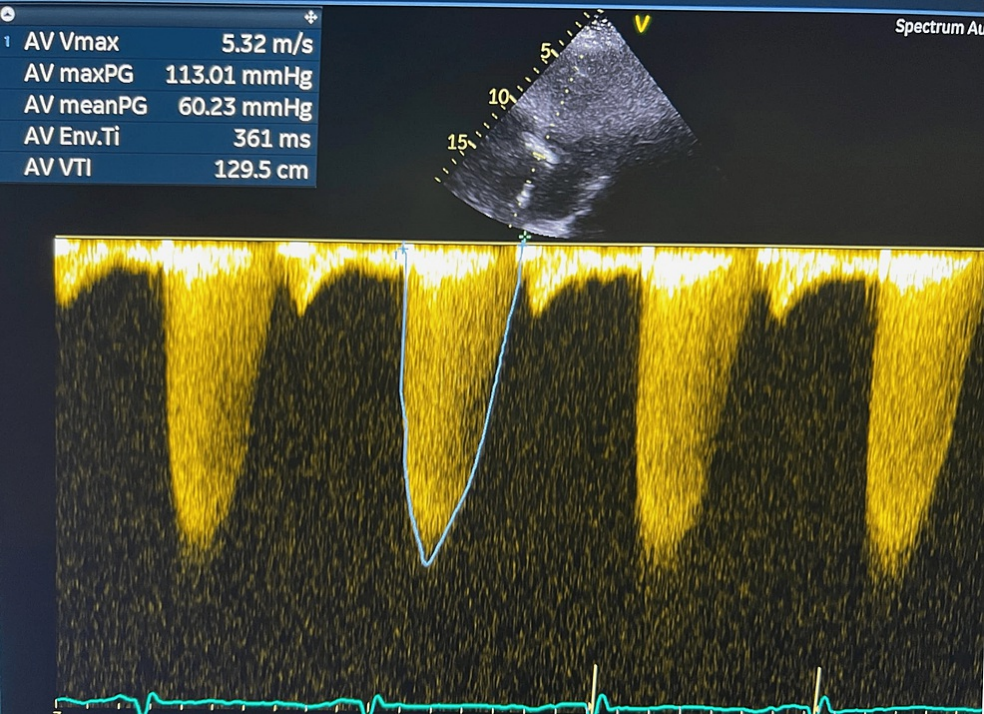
Transthoracic echocardiogram showing severe aortic stenosis with a mean aortic valve gradient of 60 mmHg and a maximum gradient of 113 mmHg
